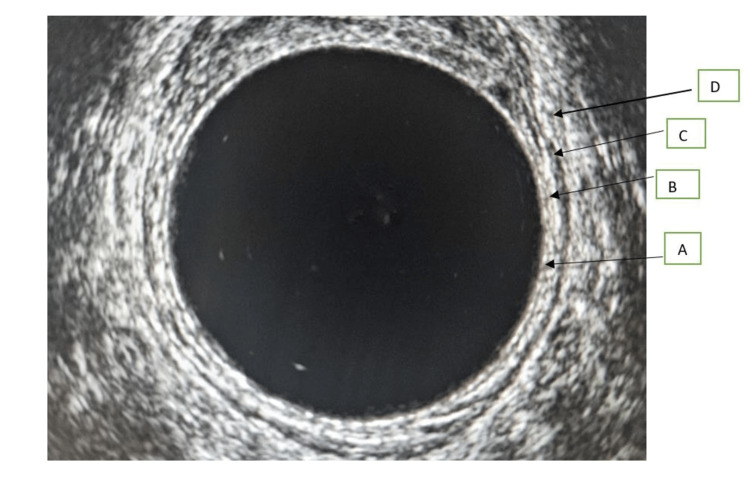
Layers of rectum visualised by endorectal ultrasound A: mucosa, B: submucosa, C: muscularis propria, D: perirectal fat. The black arrows represent the four layers of the rectum as seen on a normal endorectal ultrasound. Created by Collins O. Opara.
